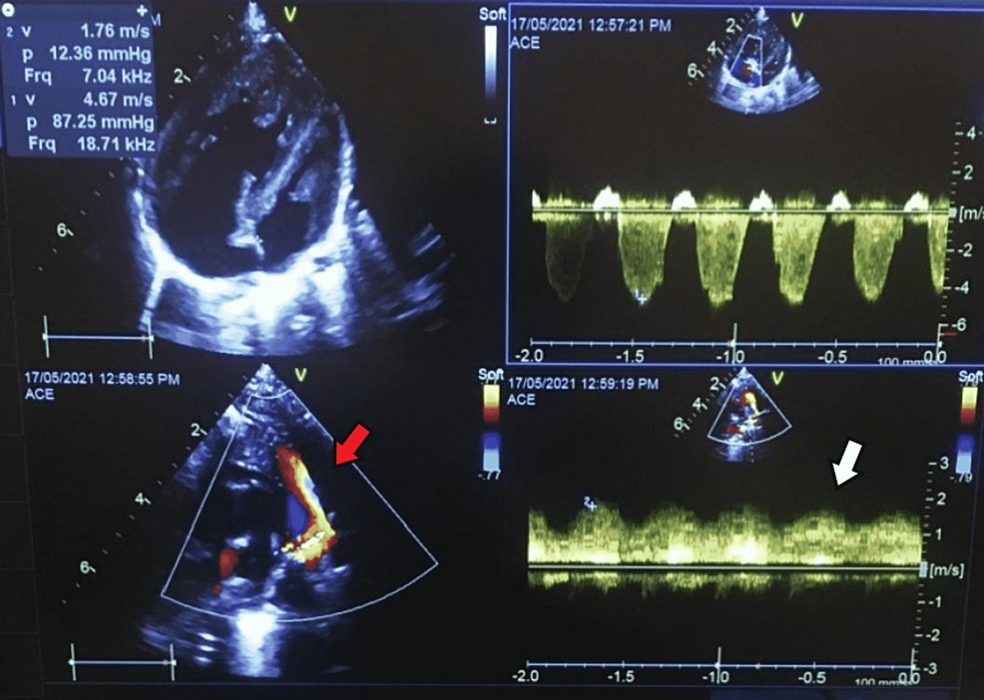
Post procedural transthoracic echocardiogram Improved flow and caliber of ascending levoatrial cardinal vein (red arrow) and peak pressure gradient was around 13mmHg (white arrow) and tricuspid regurgitation gradient was 87mmHg.
